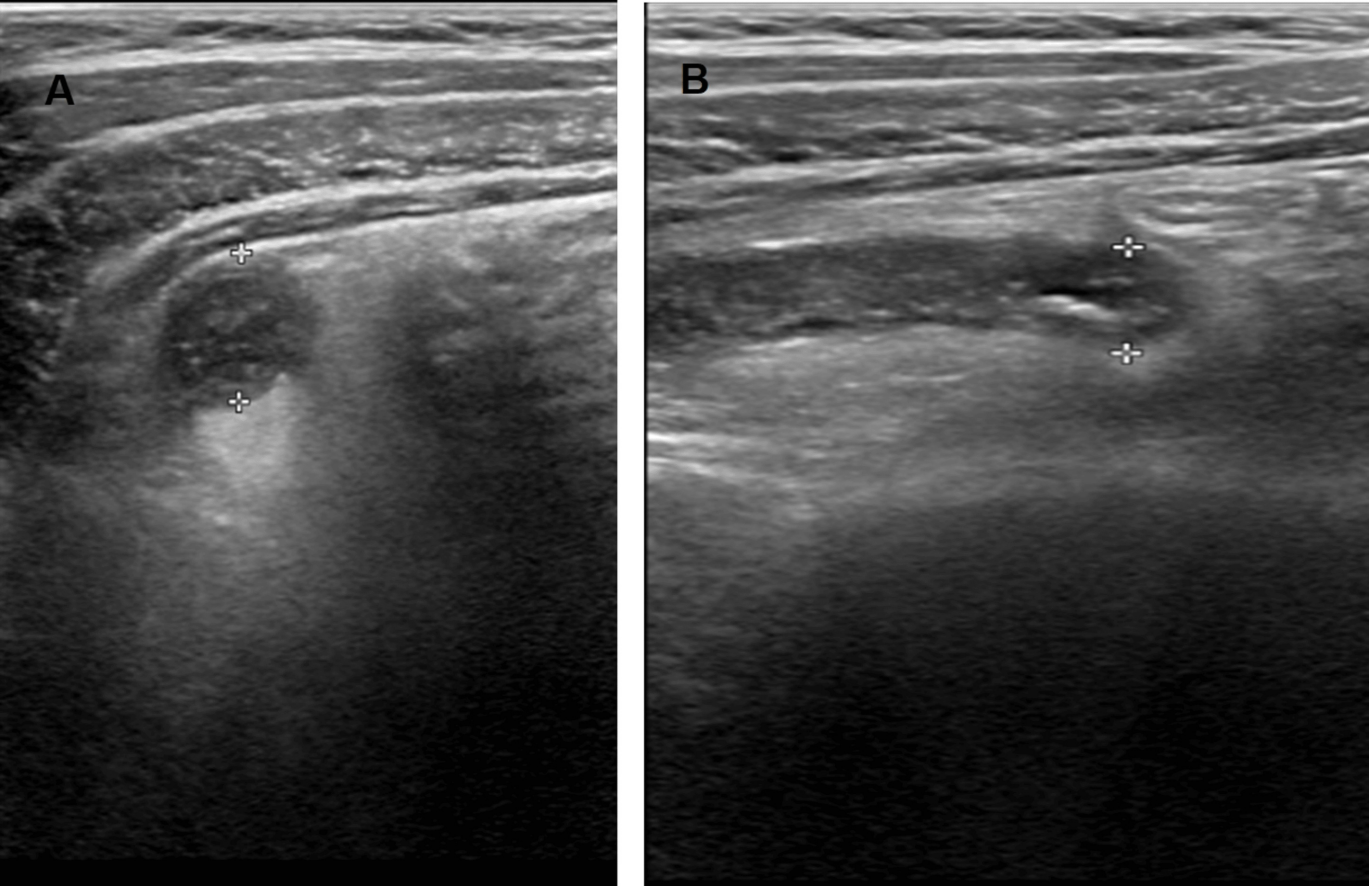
Abdominal ultrasound showing the cecal appendix with increased diameter, appendiceal wall thickening, and the presence of periappendiceal fluid (A) Transverse view demonstrating a distended appendiceal lumen with hypoechoic wall thickening. (B) Longitudinal view revealing diffuse mural thickening and adjacent fluid.
